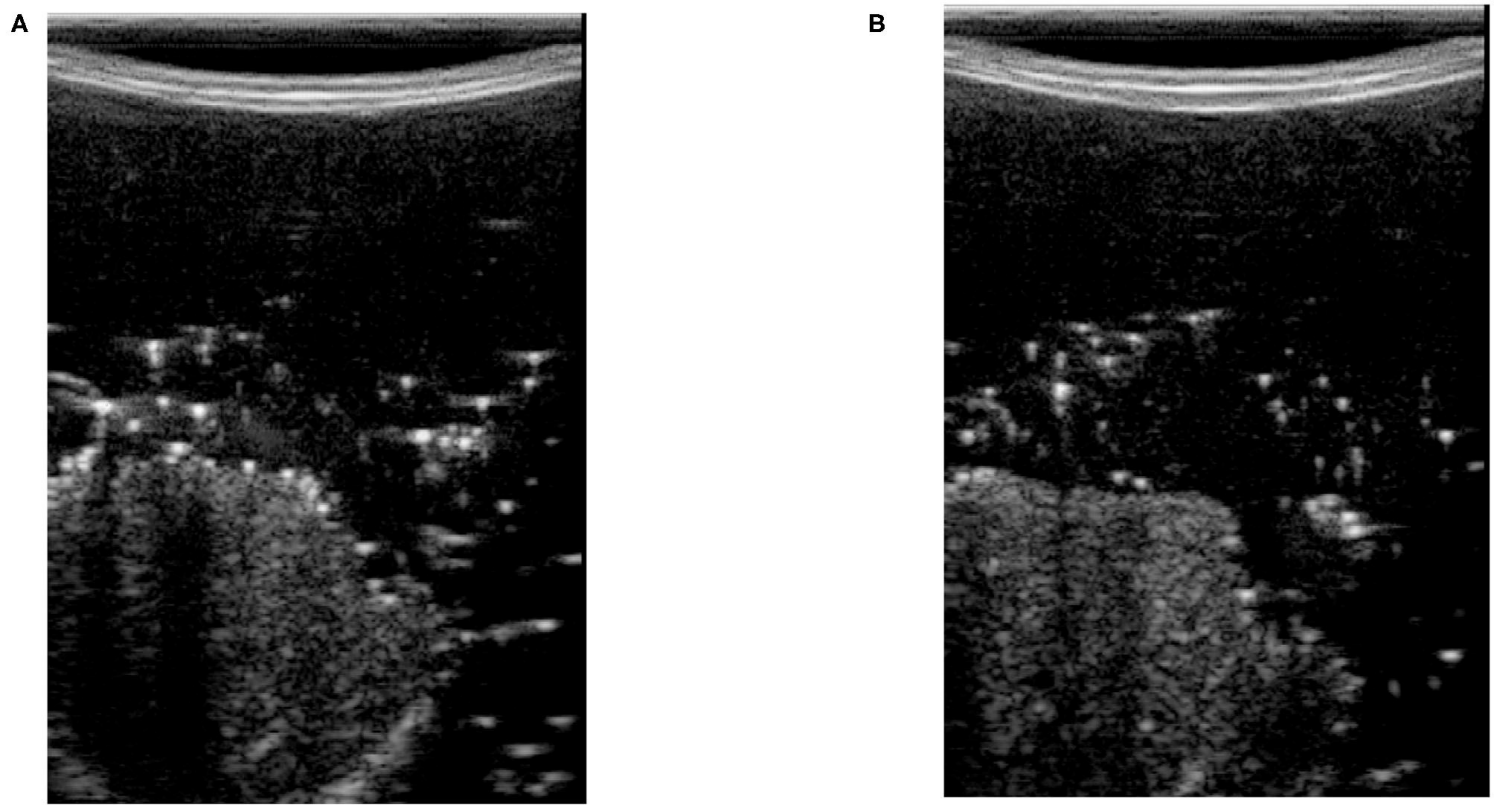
Ultrasound images with high and low levels of noise. (A) Ultrasound image with a high levels of noise. (B) Ultrasound image with a low levels of noise.
